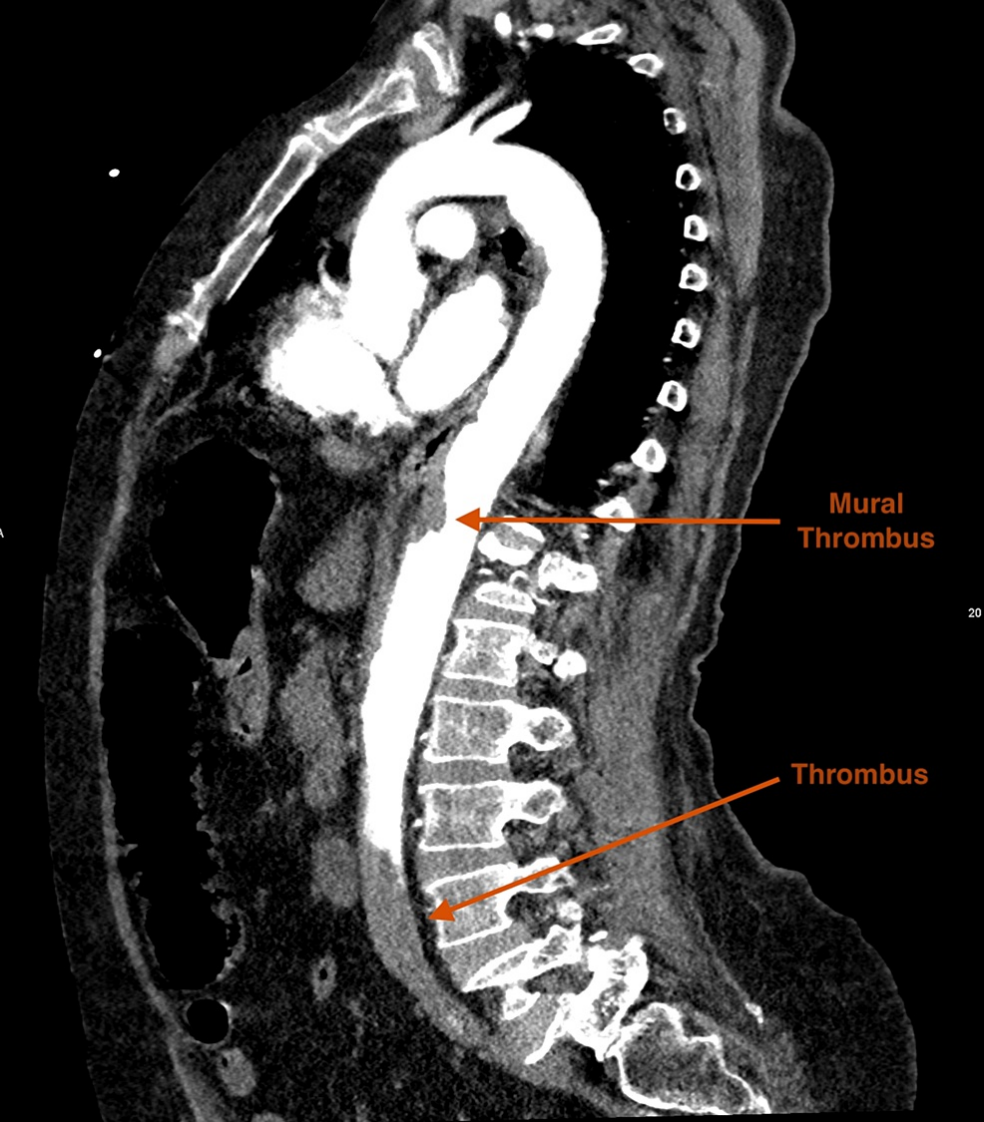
CT Angiogram - Sagittal Plane
